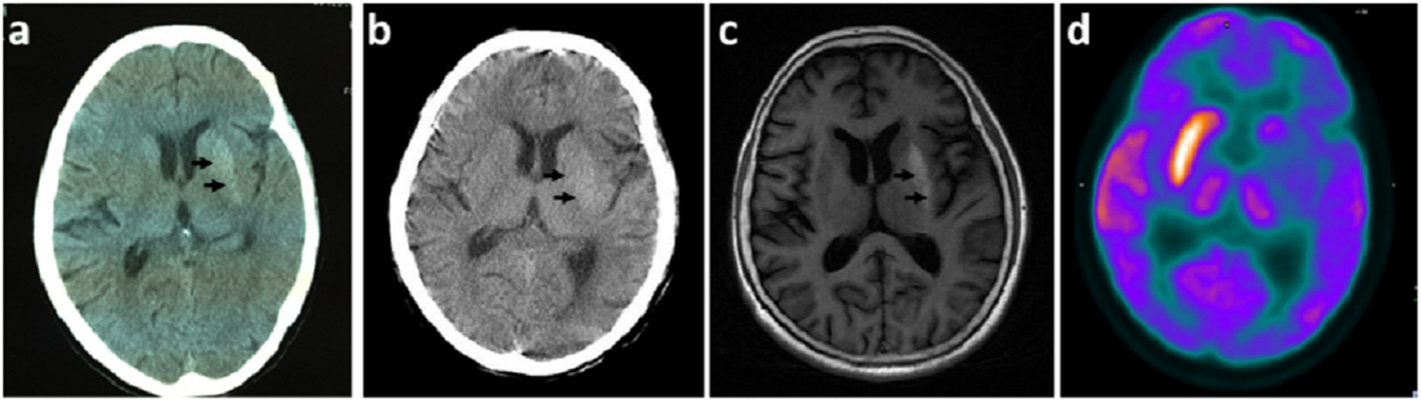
Images from patient 2. a. Brain CT (axial) the same day of onset, showing high density in the left putamen (arrow); b. Brain CT (axial) 24 days after onset, showing slight high-density in the same area (arrow), but the density decreased compared with the initial CT; c. MRI (axial) of the head 62 days after onset, demonstrating hyperintensity on T1-weighted images in the putamen (arrow); d. The FDG-PET scan (axial) 55 days after onset, revealing hypo-perfusion in the left side of the basal ganglion.
